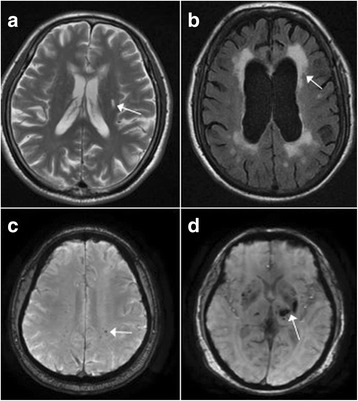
Typical images of different signs of CSVDs in PD patients. a Lacunar infarct, Insular subcortical, T2WI images; b Abnormal white matter hyperintensities, bilateral periventricular, T2-FLAIR images; c Microbleeds, bilateral parietal lobe white matter, SWI images; d Hematoma, bilateral basal ganglia and dorsal thalamus, SWI images
